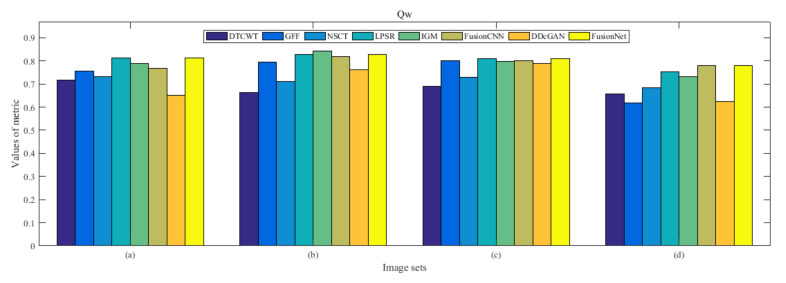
Values of $Q_{w}$ in the fused images (MRI-SPECT): (a) is composed of eight images in Figure 8, (b) is composed of eight images in Figure 9, (c) is composed of eight images in Figure 10, (d) is composed of eight images in Figure 11.
